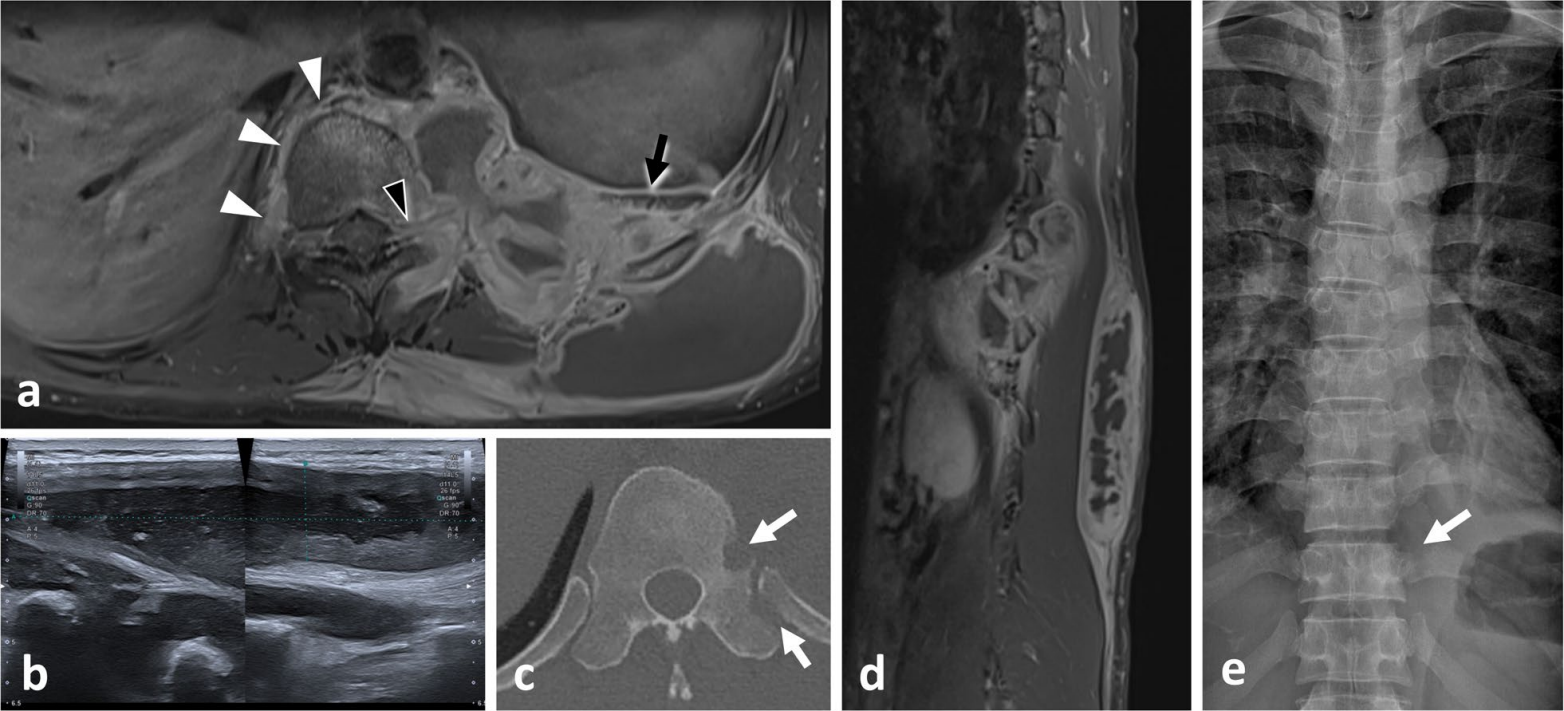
Cold abscesses* in a 37-year-old male with mild thoracic pain, weight loss and fatigue for 1 month. Axial (a) and sagittal (d) T1WI with contrast agent show large left paraspinal abscesses, which extend in both hypaxial and epaxial (superficial and deep) regions. Abscesses extend along the vertebral body to the contralateral side (white arrowheads) and into the left vertebral foramen (black arrowhead). Axial CT scan reconstruction (c) and anteroposterior radiograph (e) show septic arthritis of the costovertebral joint and spondylitis (white arrows). Anteroposterior radiograph shows the left hypaxial mass along the left side of the side (black arrows). As the lesion was superficial, US was used for percutaneous guided biopsy (b). US shows a hypoechoic mass with posterior acoustic enhancement
